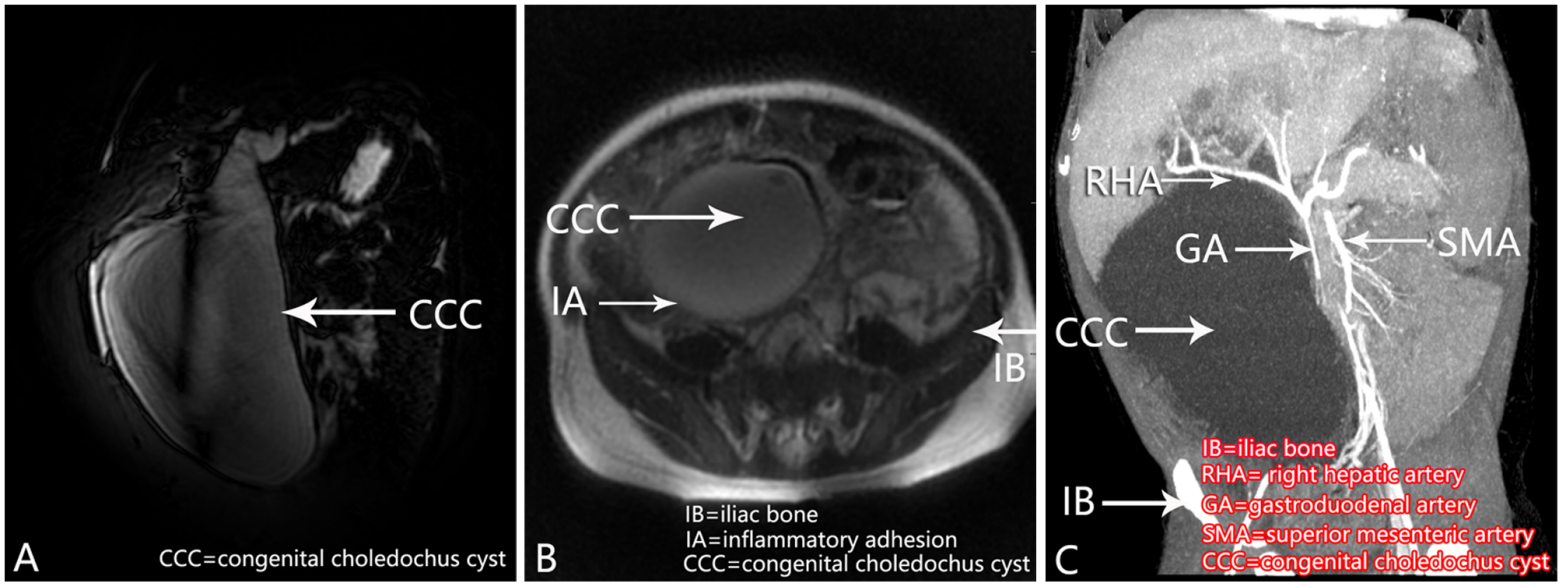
A 1-year old female with cyst descension into the introitus pelvis. A, Pancreatic and common channel was not detected in MRCP. B, Axis T2WI imaging showed the cyst descension into introitus pelvis. Inflammatory adhesion can be found in the lower-right side of the cyst. C, contrast-enhanced multislice spiral computed tomography (multiplanar reformation imaging) showed the cyst occupies large intra-abdominal space, displacement of vessels, modified the pelvic and duodenal anatomy.
